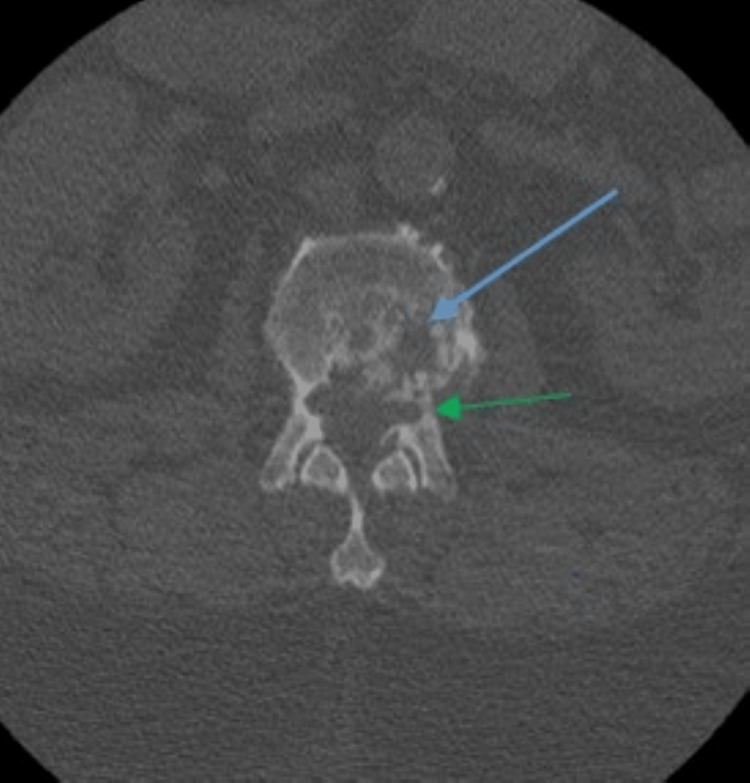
Axial computed tomography image shows a heterogeneous lytic bone erosion lesion involving the posterior left L2 vertebral body (blue arrow) with extension to the left posterior elements (green arrow).
